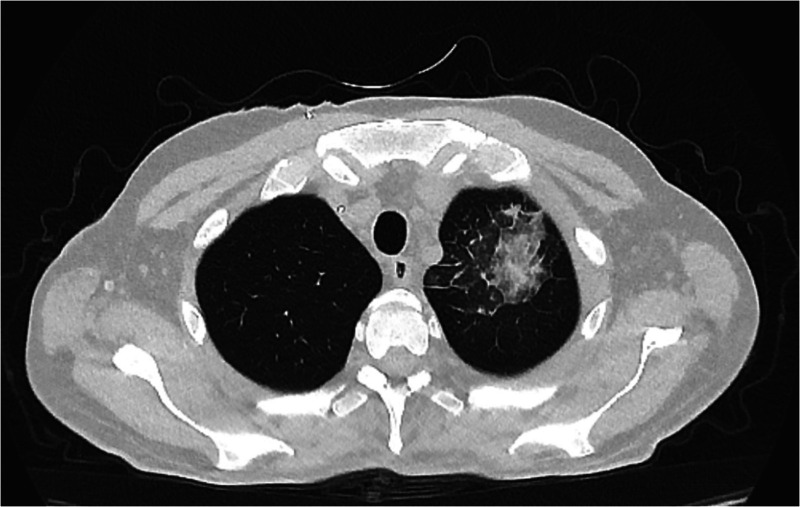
Computed tomography of the chest (axial image).
